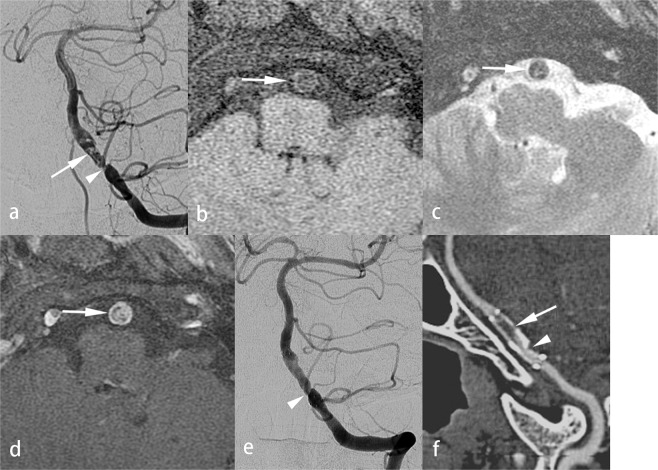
Hard lesion in a patient with dissection in atherosclerosis (DA). A 54-year-old man was a heavy smoker and had a history of neck massage. He suffered from stroke with right hemiparesis in the past 1 year and had recurrent acute diplopia and dysarthria 1 month ago. Left vertebral angiogram showed a 90% stenotic lesion of the left distal vertebral artery (a, arrowhead) and disrupted intimal flaps (a, arrow). VW-MRI showed low signal intensity on fat-suppressed T1WI (b, arrow) and T2WI (c, arrow) and strong enhancement on contrast-enhanced fat-suppressed T1WI (d, arrow). PTAS was done and revealed a predilatation pressure (PP) of 6.5 atm. A 4 × 20 mm Wingspan stent was deployed in the left distal vertebral artery to basilar artery (e). About 30% residual stenosis was noted on the control angiogram (e, arrow). A 2-year follow-up CTA, curved multi-planar reconstruction, revealed 90% in-stent restenosis (f, arrow) and 30% residual stenosis of the proximal stent (f, arrowhead).
